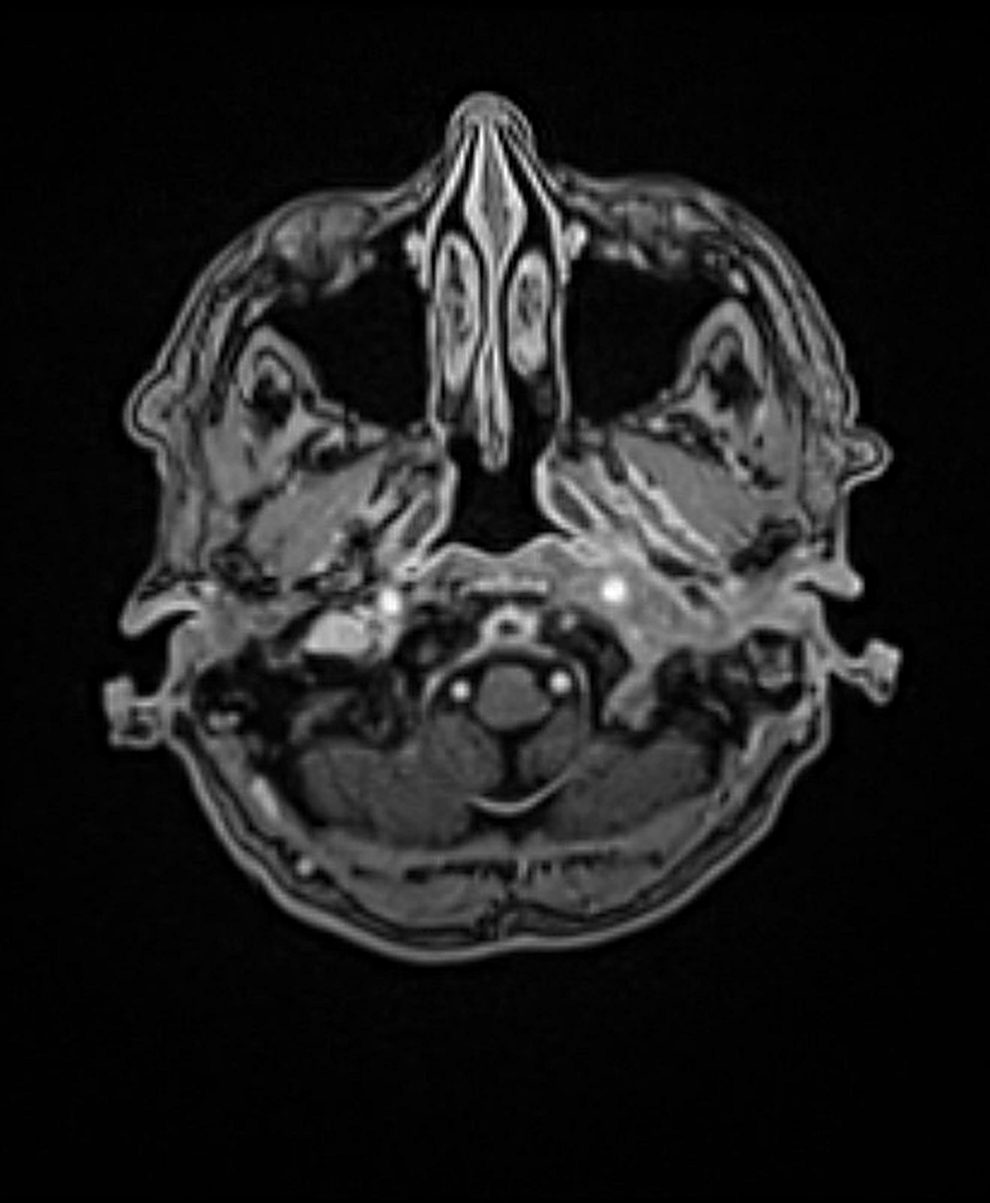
MRI examination after 6 months (TSBO)
